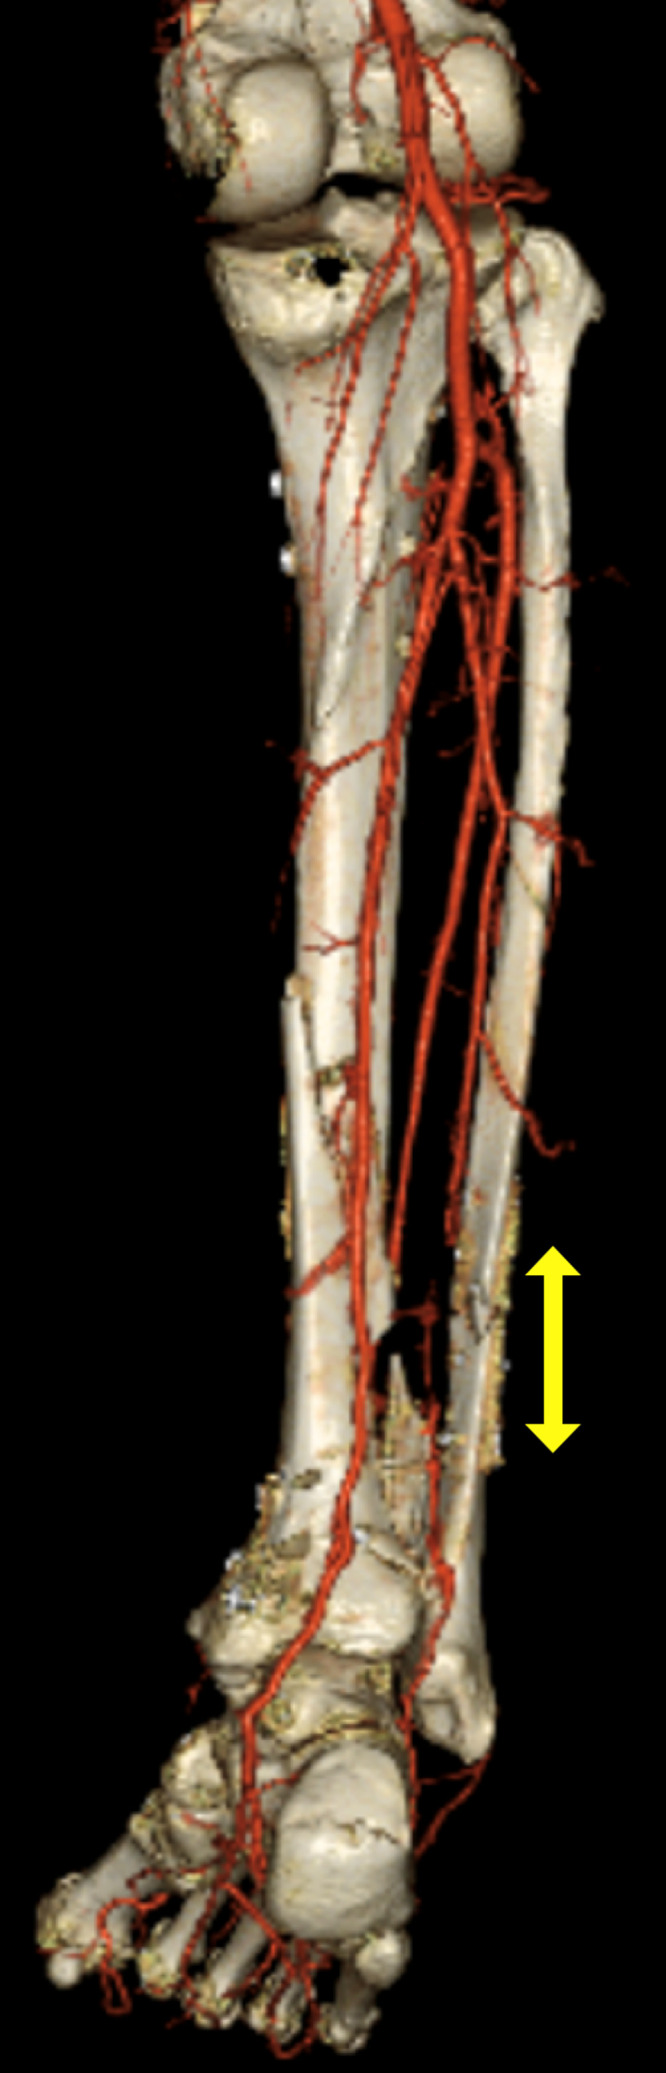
Contrast-enhanced CT at the time of transfer to our hospital. Although the anterior and posterior tibial arteries were intact, the peroneal artery was interrupted at the fracture site (yellow arrow).
